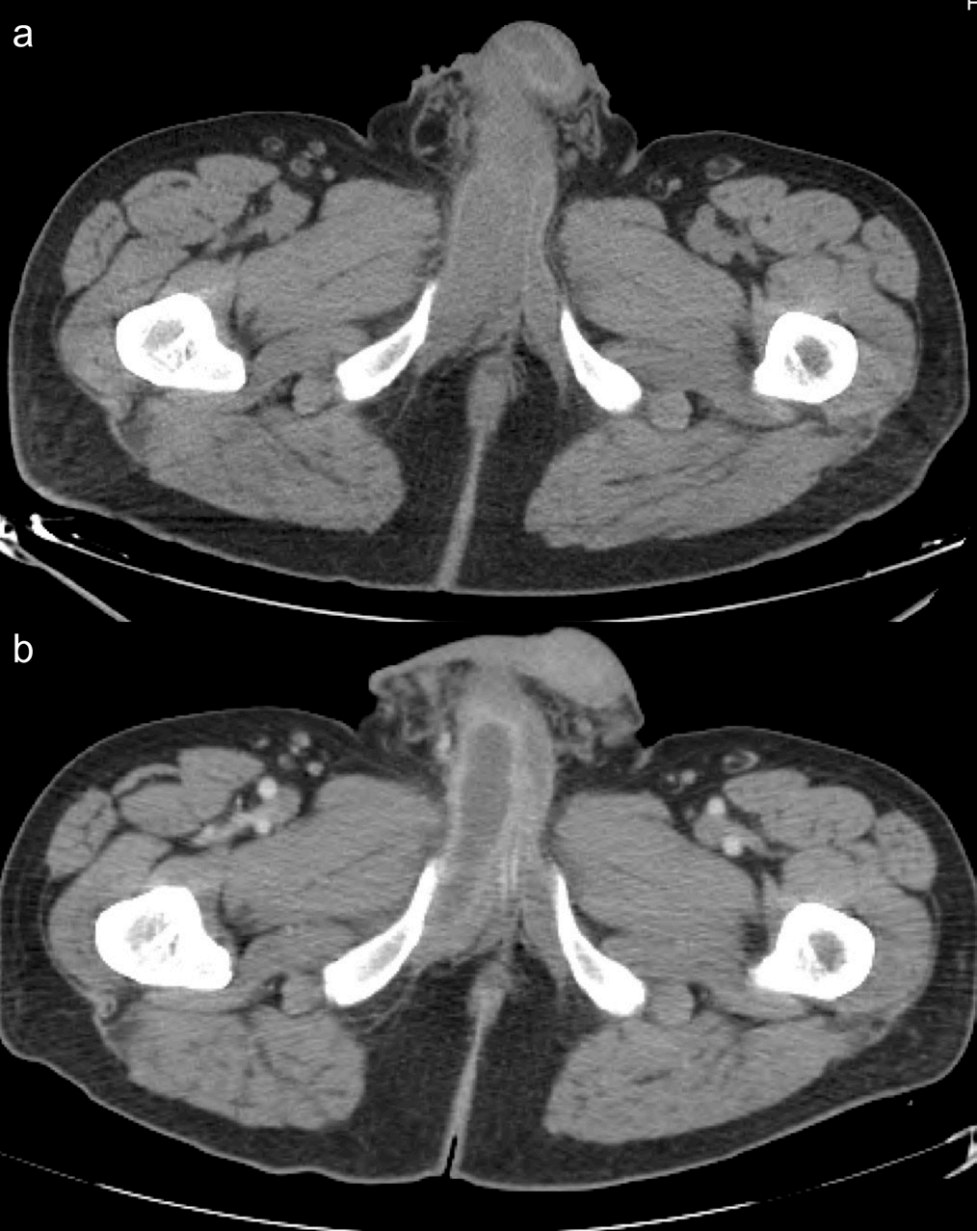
Non-contrast CT (1a) shows an asymmetrical enlargement of the proximal segment of the right corpus cavernosum. In contrast-enhanced CT (1b), the mass remains hypodense with a hyperdense rim of enhancement, causing mass effect on the left corpus cavernosum.
